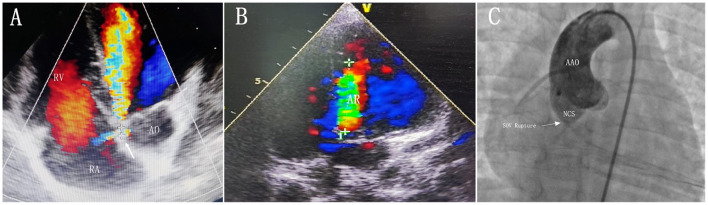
(A) Transthoracic echocardiogram obtained in the five-chamber view showing that the SOV rupture occurr in the non-coronary sinus and rupture into the right atrium. (B) Transthoracic echocardiogram obtained in the apical long axis view showing a mild-to-moderate aortic regurgitation. (C) Aortography showing significant shunting between NCS and the right atrium. The defect diameter of 3 mm was measured by aortography. AR, aortic regurgitation; AO, aorta; AAO, ascending aorta; RA, right atrium; RV, right ventricle; NCS, non-coronary sinus of Valsalva; SOV rupture, Sinus of Valsalva rupture.
